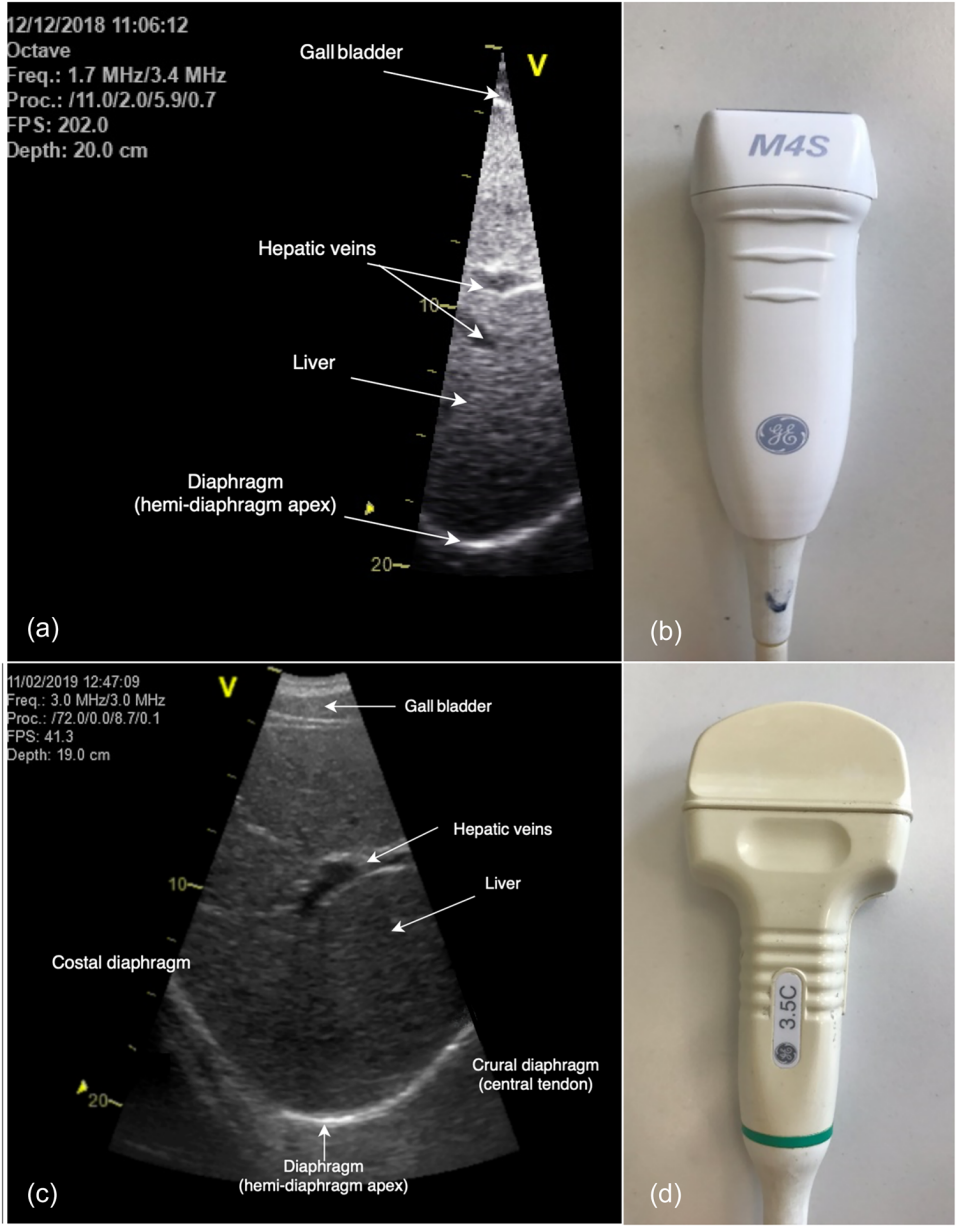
Representative B‐mode ultrasound images of the right crural hemidiaphragm at relaxation volume in a female participant. Images were obtained using a phased‐array transducer (a, b) and a curved‐array transducer (c, d).
